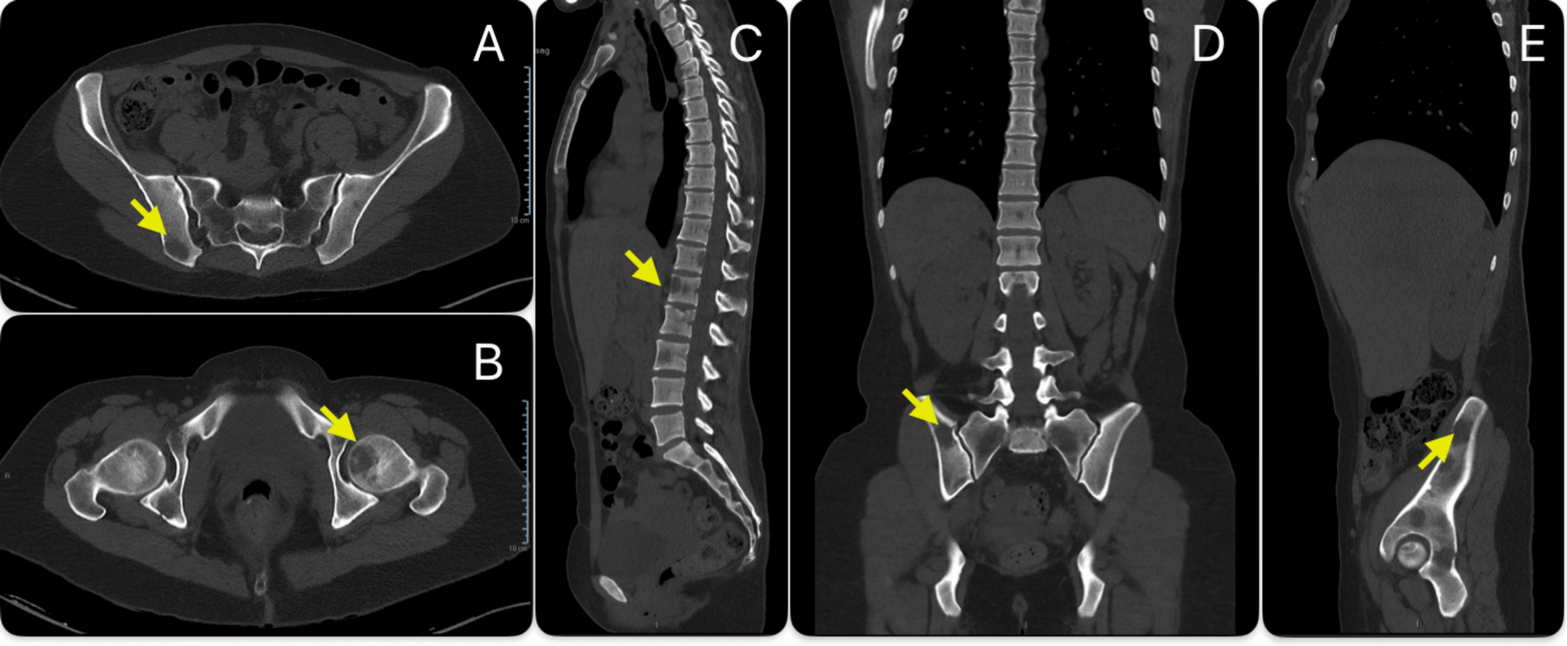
Vertebral spine and pelvic computed tomography scan Numerous lytic lesions were observed in the vertebral spine and pelvis (transverse section of pelvic CT scan – A, B; sagittal section of vertebral and pelvic CT scan – C, E; coronal section of vertebral and pelvic CT scan – D).
